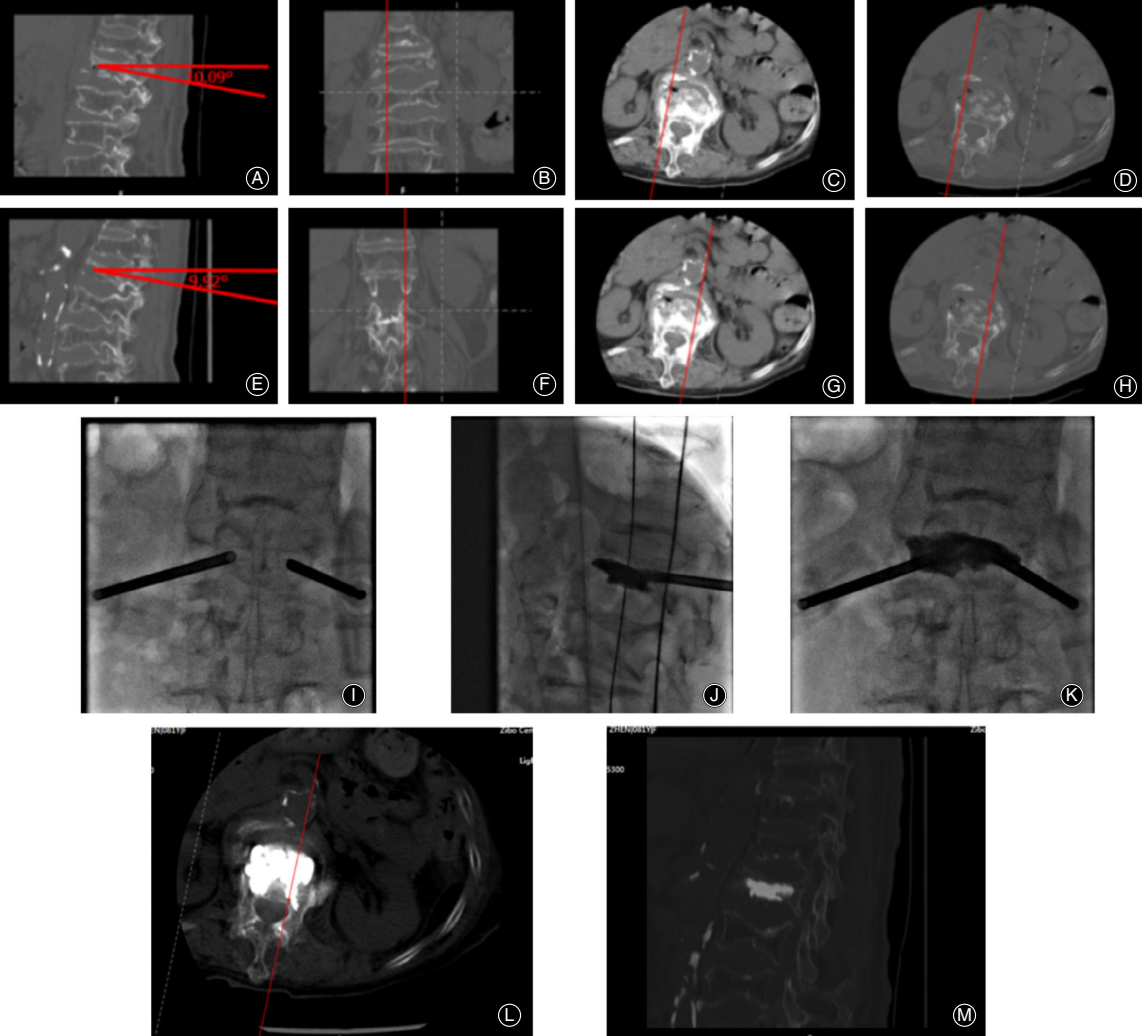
PVP surgical procedure with side‐opening cannula. (A–D) The angle and position of the right side of the vertebral body are determined based on the CT scan results. (E–H) The angle and position of the left side of the vertebral body are determined based on the CT scan results. (I) An anteroposterior X‐ray film after implantation of the cannulas on both sides. (J–K) The bone cements were gradually injected into both sides, and the cannulas were rotated to adjust the direction of the bone cement outlet to ensure uniform distribution in the vertebral body. The anteroposterior and lateral X‐ray film after bone cement injection. (L‐M) CT scan results showed that the bone cement was evenly distributed without leakage.
